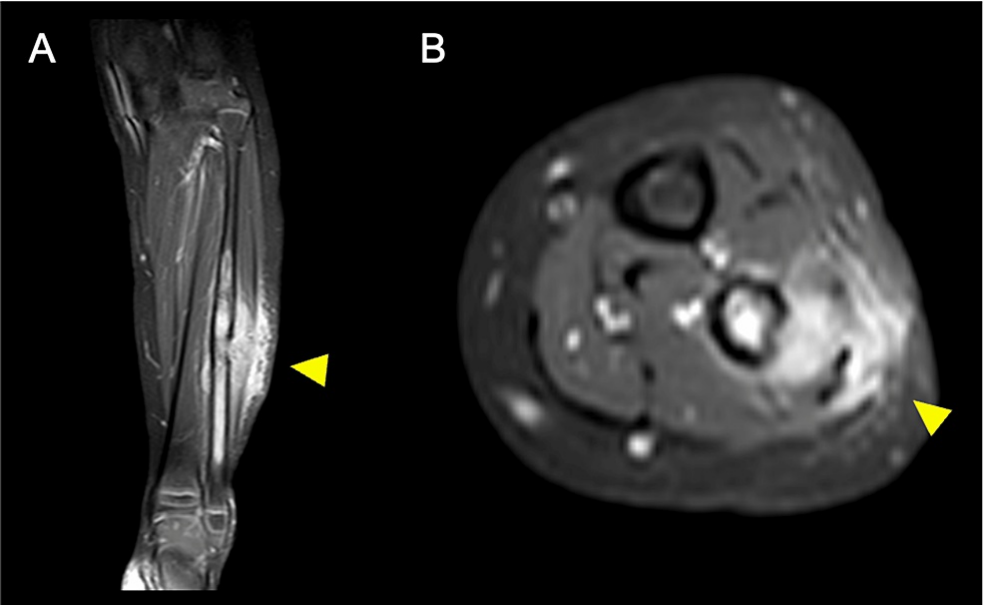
Contrast-enhanced fat-suppressed T1-weighted image of the left lower leg There is a contrast area from the diaphysis of the left fibula to the distal end of the diaphysis. (A) Coronal plane; (B) Axial plane
